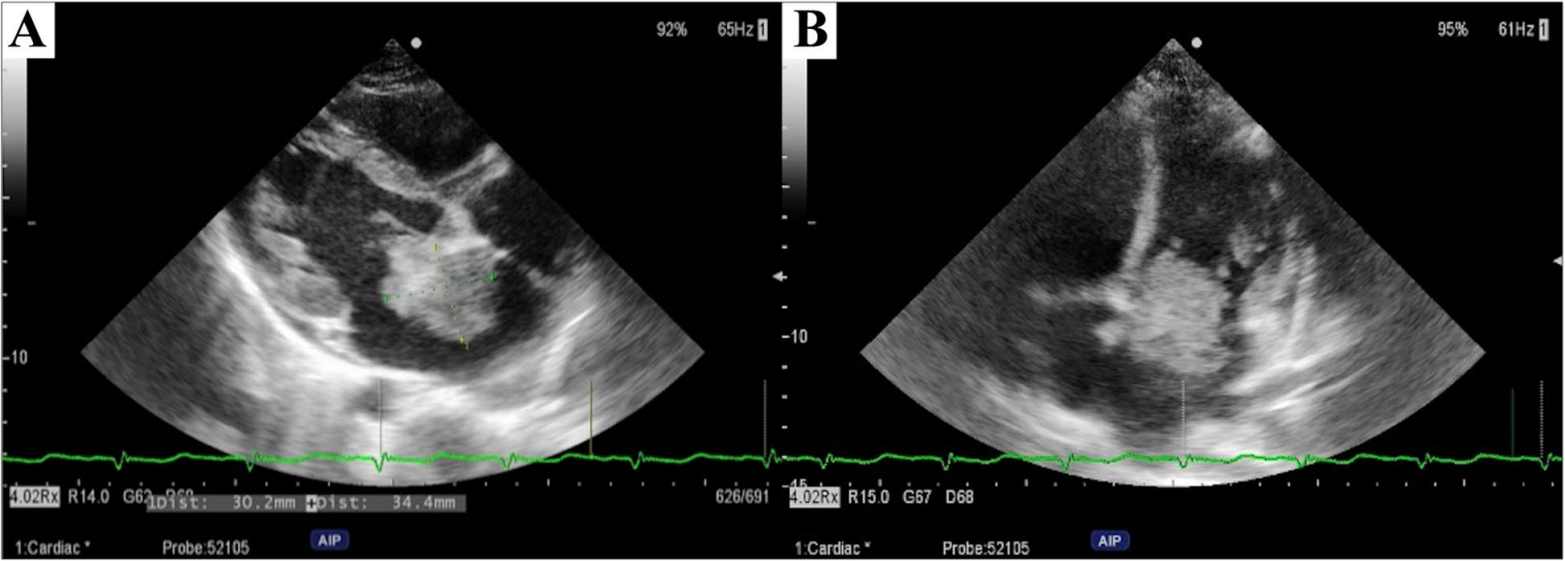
Preoperative transthoracic echocardiographic images. A Transthoracic echocardiography shows a tumor arising at the atrial septum in the left atrium, measuring 30 × 34 mm. B The cardiac tumor is shown invaginated into the mitral valve during diastole
